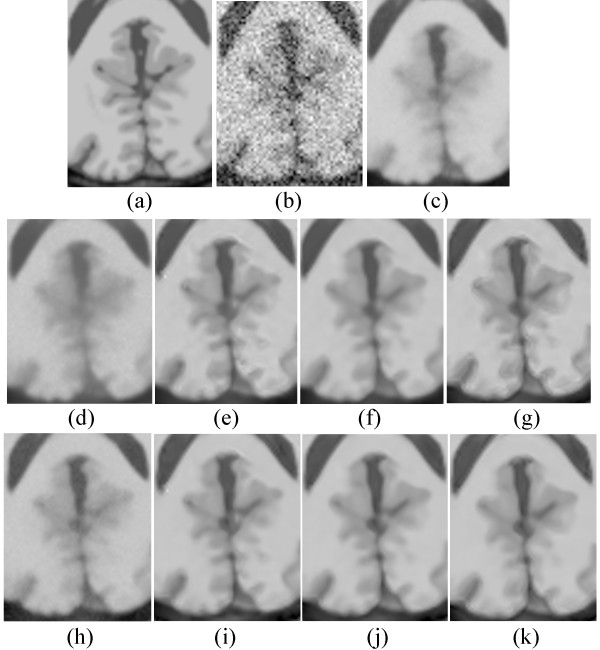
Comparison of experiment results on T1-weighted MRI. (a) original MRI, (b) noisy MRI with 17% Rician noise, (c) results of the original NLM filter, (d) results of the UNLM with squared magnitude transformation, (e) results of the PSNLM1 with median filter, (f) results of the PSNLM1 with Gaussian filter, (g) results of the PSNLM1 with anisotropic filter, (h) results of the UNLM with VST, (i) results of the PSNLM2 with median filter, (j) results of the PSNLM2 with Gaussian filter, (k) results of the PSNLM2 with anisotropic filter.
